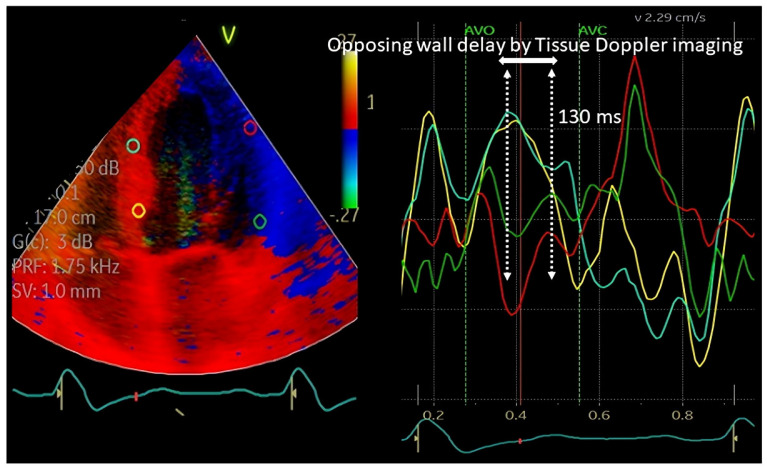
Tissues Doppler Imaging. Regions of interest are placed on the left ventricle in a four-chamber apical view, and delay in the peak velocity of the opposing walls is estimated. Based on the literature, a delay of >65 ms is regarded as a significant delay.
